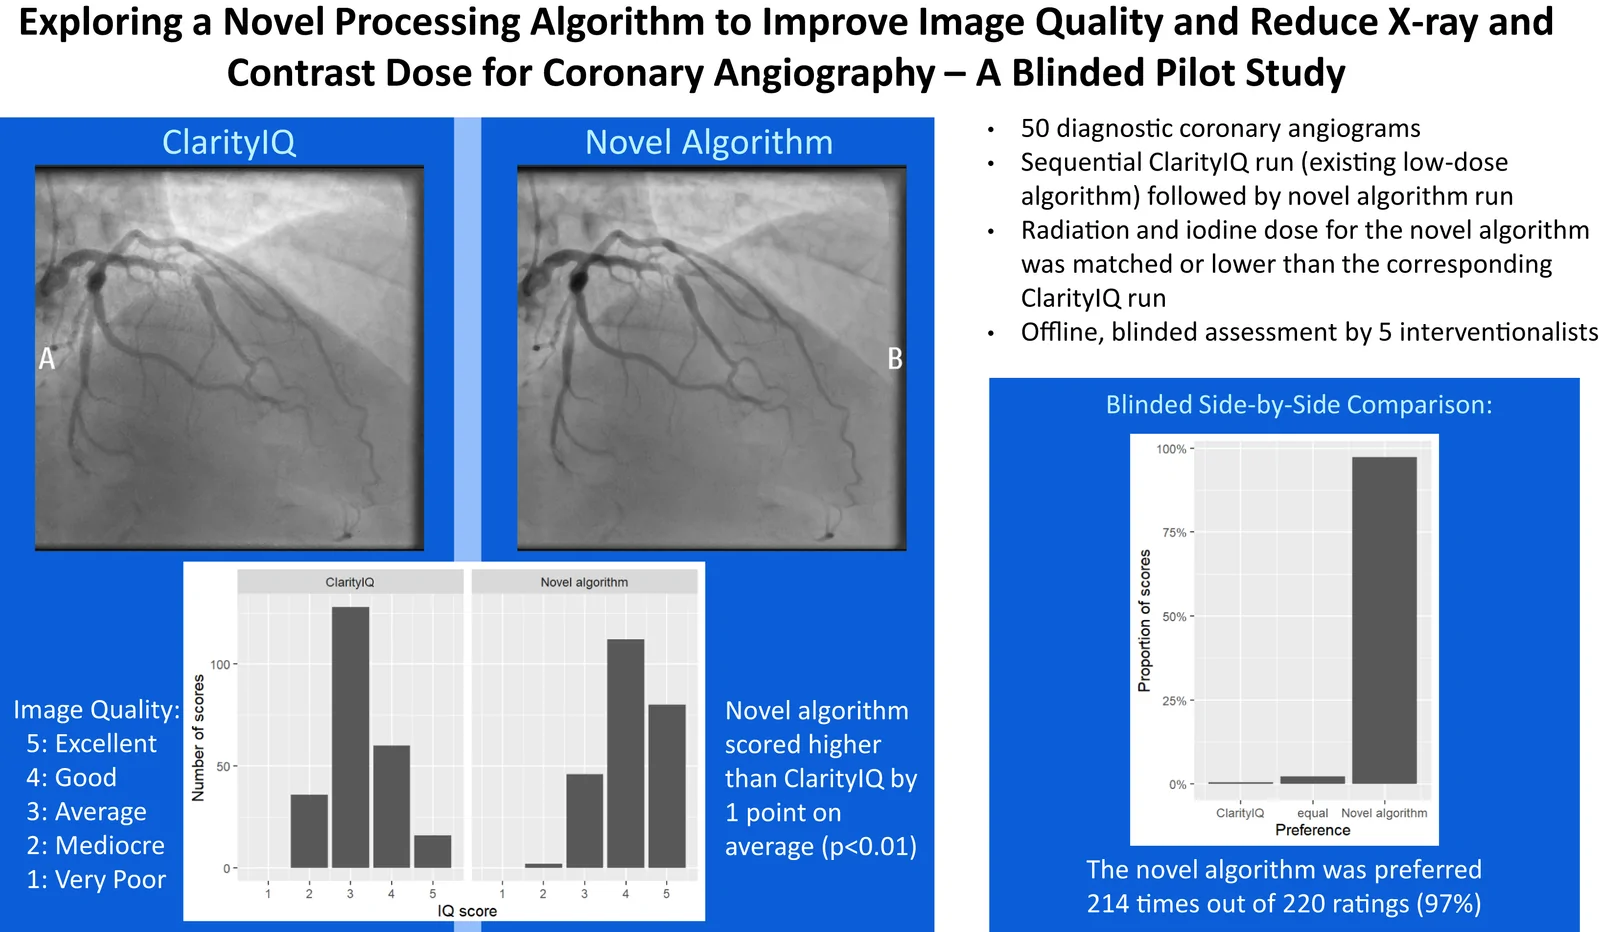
Exploring a novel processing algorithm to improve image quality and reduce x-ray and contrast dose for coronary angiography – a blinded pilot study.
